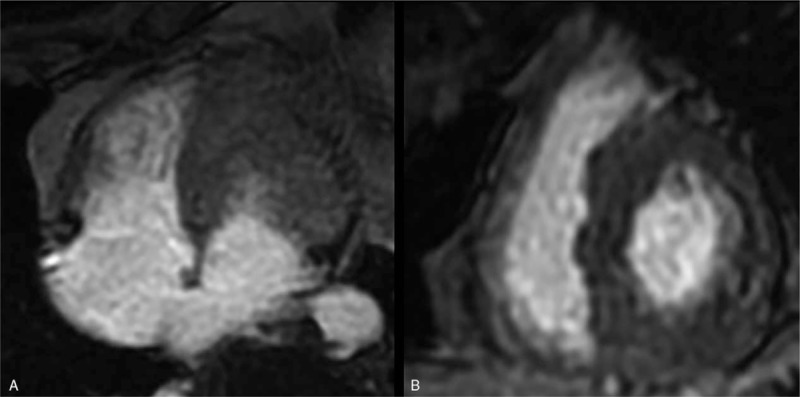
(A repeated MRI after 17 months): There was no myocardial area of late post-contrast enhancement detected on images in the four-chamber view (A) and short axis (B). ^∗^MRI = magnetic resonance imaging.
